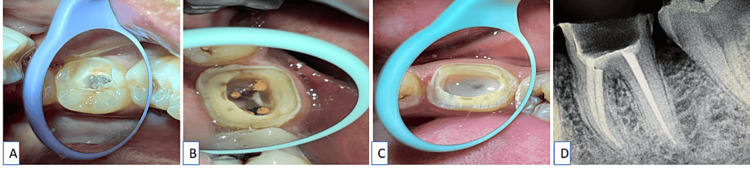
(A) Previous root canal treatment on tooth number 36; (B) tooth preparation performed on tooth number 36; (C) glass ionomer cement to seal the pulpal floor; (D) radiograph taken following tooth preparation with tooth number 36
